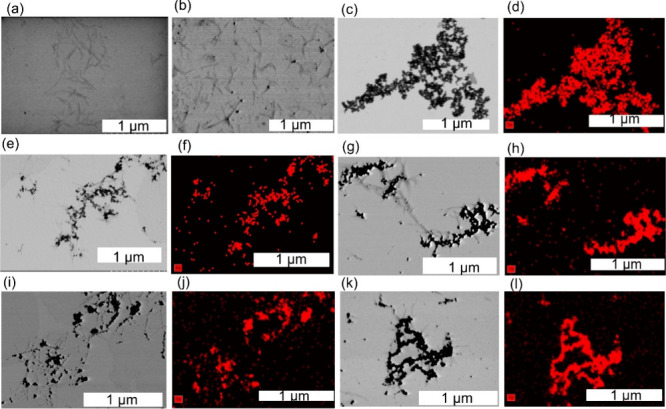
Bright-field STEM images and corresponding energy-dispersive X-ray
